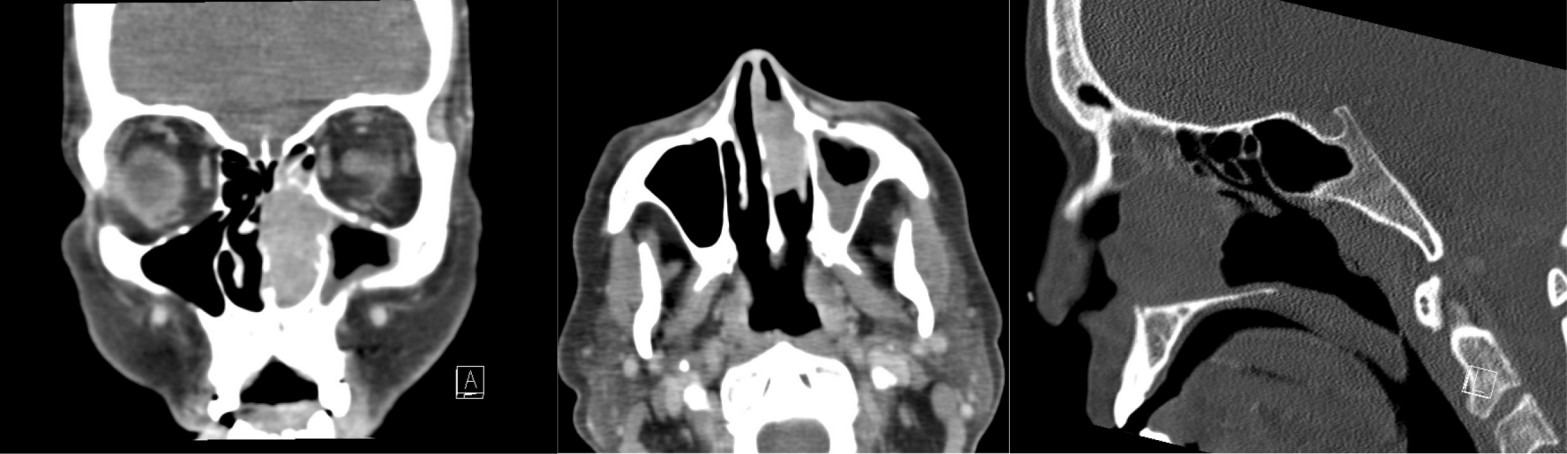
CT sinus in 3 different views of the sinonasal mass on presentation.
